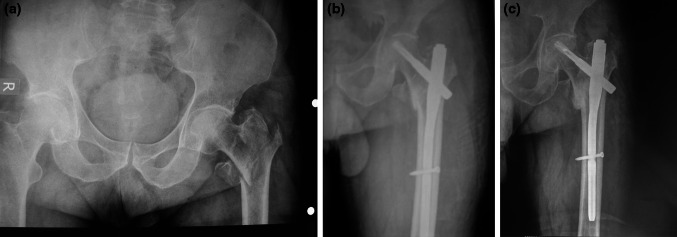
Seventy-six-year-old female patient sustaining an isolated and closed 31-A2 fracture at the *left* side (a) after a simple fall. Postoperative X-ray after closed reduction and internal fixation with a PFNA (b). Final anteroposterior hip radiograph at the 12-month follow-up (c)
